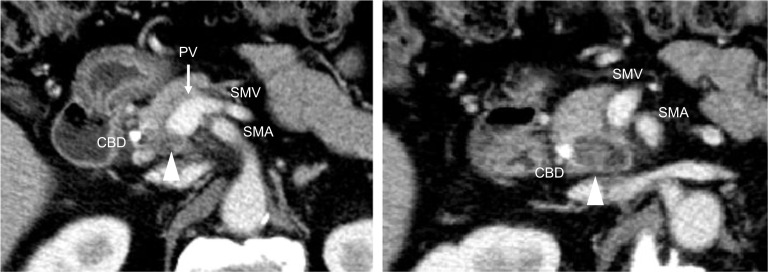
Enhanced computed tomography (CT) after neoadjuvant chemoradiation. Abdominal CT demonstrates reduction of tumor, 20 mm (arrowhead), with clinical partial response; the boundary with the superior mesenteric vein and portal vein (arrow) is clear
